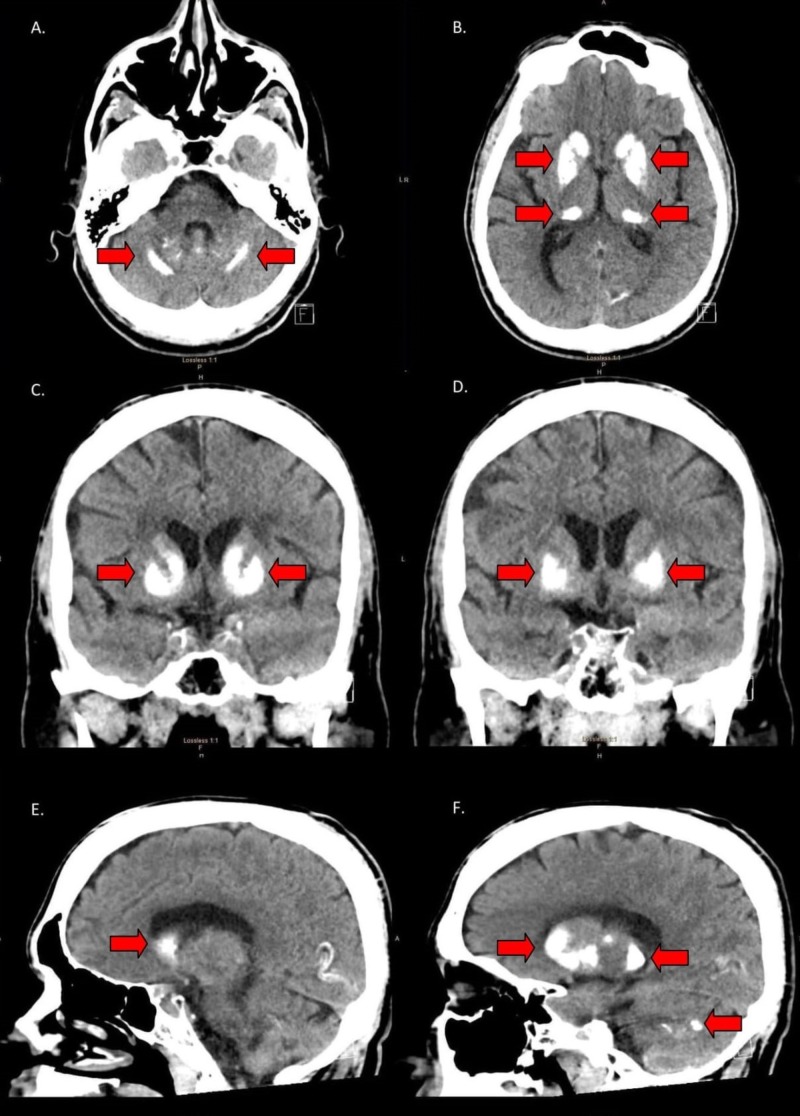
CT images displaying calcifications of the basal ganglia and cerebellum A. Axial CT image showing bilateral, linear calcifications of the cerebellum. B. Axial CT image showing bilateral calcifications of the putamen and the head and tail of the caudate nucleus. C. Coronal CT image showing bilateral calcifications of the caudate nucleus and putamen. D. Coronal CT image showing bilateral calcifications of the putamen. E. Sagittal CT image showing calcification of the head of the caudate nucleus. F. Sagittal CT image showing calcifications throughout the basal ganglia and cerebellum. CT: computed tomography
